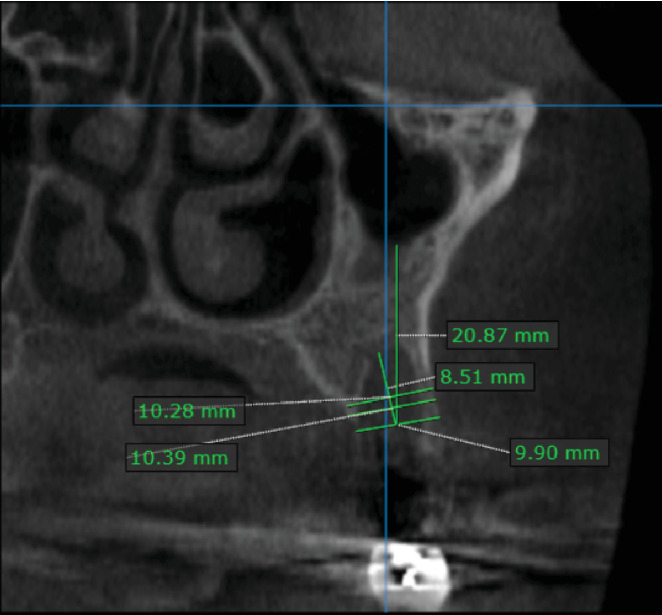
The radiographic measurements immediately after the surgical procedures.
